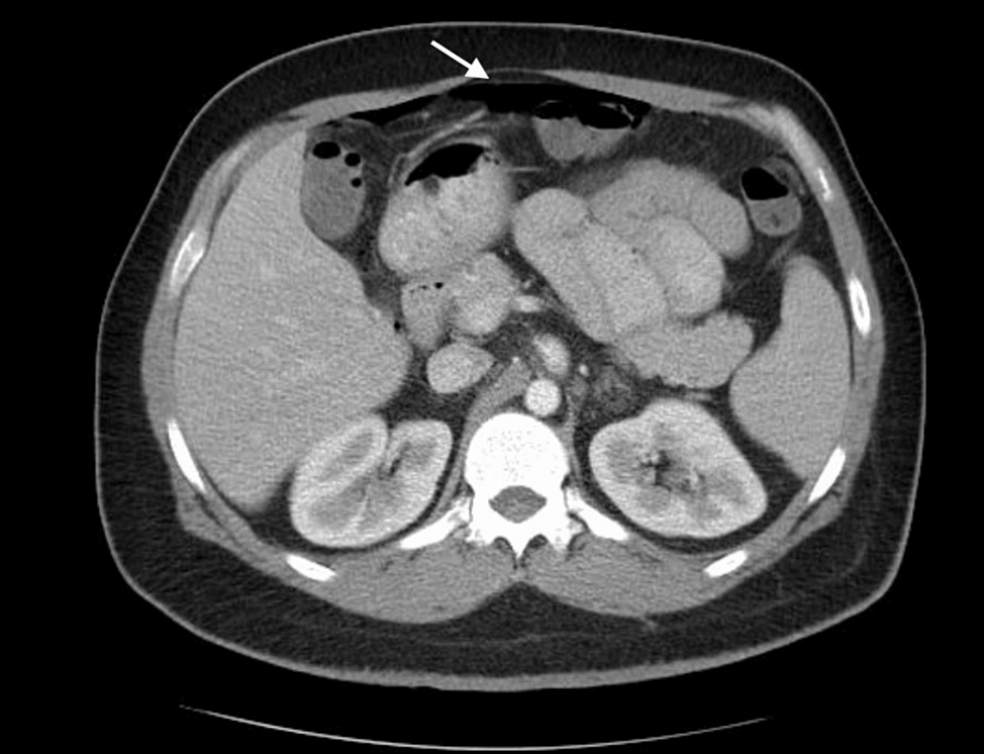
CT scan reveals free air in the abdomen (arrow)
